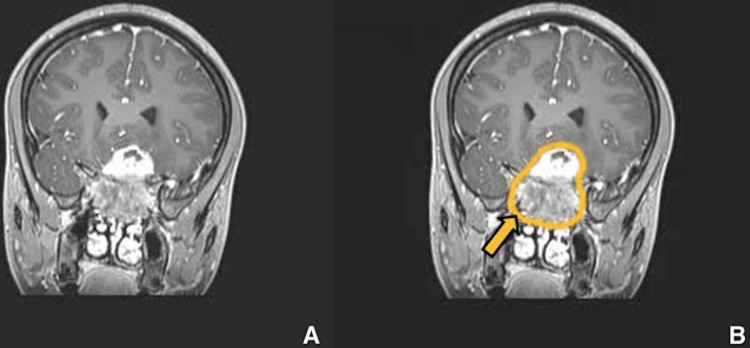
Preoperative imaging for patient 2. Contrast-enhanced T1-weighted coronal image showing sellar, suprasellar lesion with cavernous sinus invasion. (A) Without ROI and (B) with ROI.
